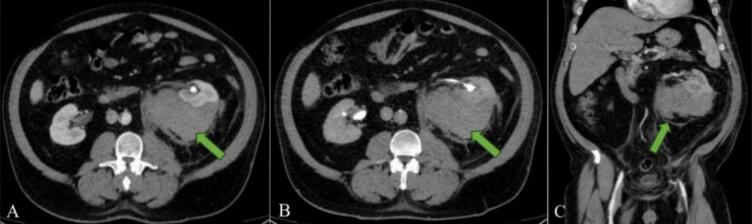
Contrast-enhanced abdominal CT scan demonstrating a large left perinephric hematoma (green arrow) exerting a mass effect on the left kidney. The delayed phase (B) reveals anterior displacement of the left kidney and pelvicalyceal system, with no evidence of contrast extravasation. (For interpretation of the references to colour in this figure legend, the reader is referred to the web version of this article.)
